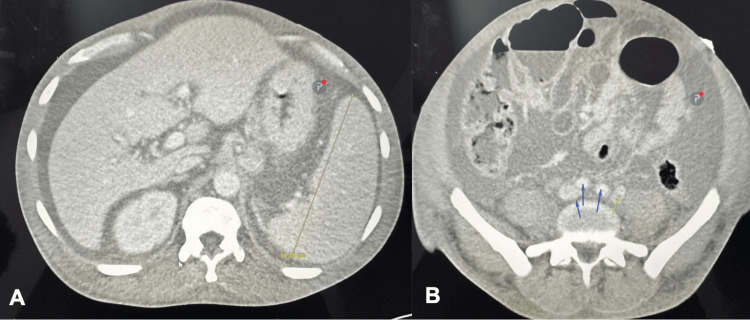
Axial CT scans of the abdomen displaying (A) hepatosplenomegaly, as demarked by the yellow line, and ascites. Imaging further reveals (B) retroperitoneal lymphadenopathy, highlighted by the arrows.
